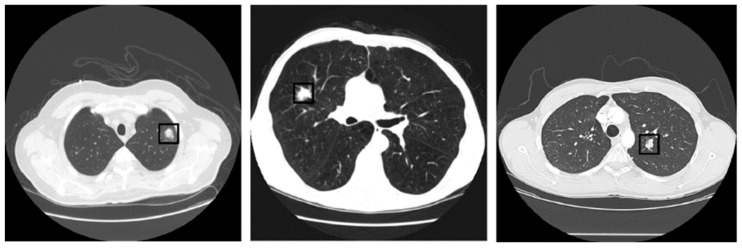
Categories of lung nodules in a CT scan; benign, primary malignant, and metastatic malignant (from left to right).
